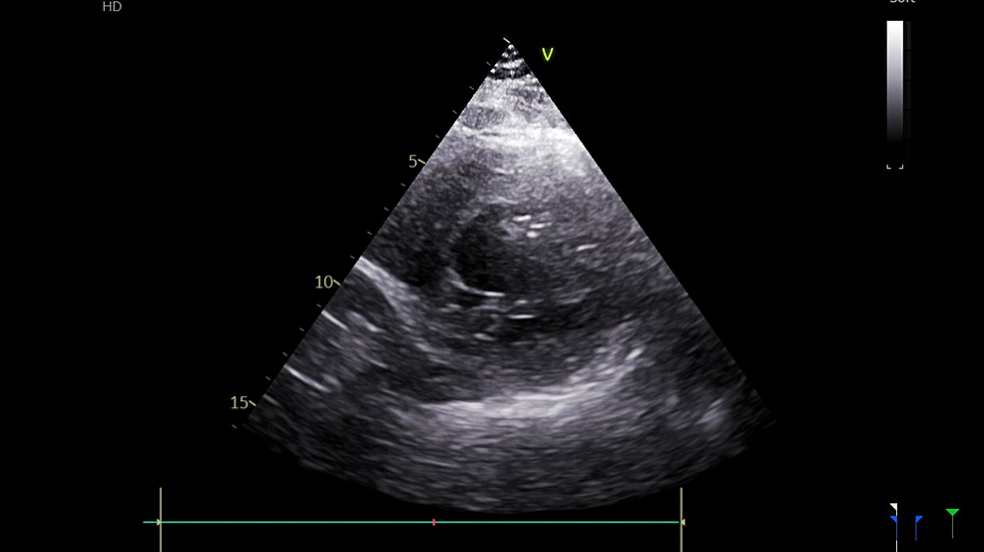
Echocardiogram in the diastolic phase.
